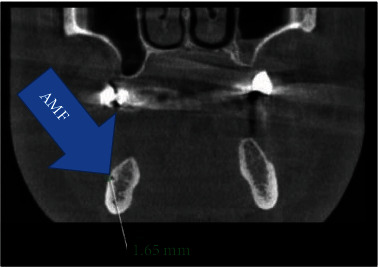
Right AMF at coronal CBCT.
